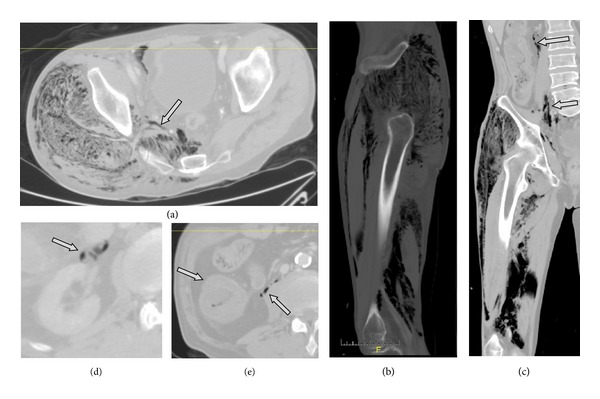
Preoperative CT scan. (a) shows an axial slice of the pelvis a little proximally from the acetabula with a massive infestation of the gluteal muscles and the intrusion of the infection into the retroperitoneal space in the true pelvis though the sciatic notch (arrow). (b) shows a sagittal reconstruction slice with extensive infestation of the whole circumference of the thigh. Gluteal musculature, the extensor muscles (quadriceps and rectus), and hamstrings are affected. (c) shows a coronary reconstruction with again massive affection of the thigh. It also indicates the disseminated infestation of the retroperitoneum with evidence of gas (arrows). (d) shows gas next to the vascular pedicle of the right kidney (arrow). (e) shows the thickened ascending colon (left arrow) and again retroperitoneal gas (right arrow).
